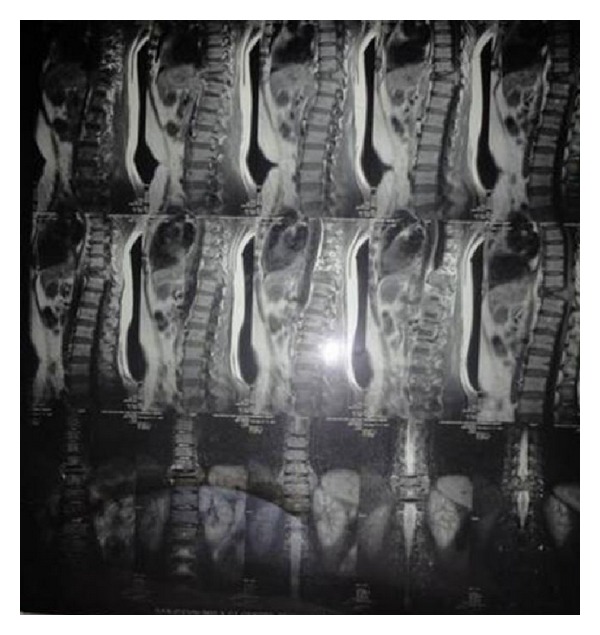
MRI of dorsolumbar spine showing complete marrow replacement of vertebral body and posterior elements with associated homogenously enhanced soft tissue component in adjacent pre- and paravertebral space and in ventral and dorsal epidural spaces leading to severe cord compression and spinal canal stenosis.
